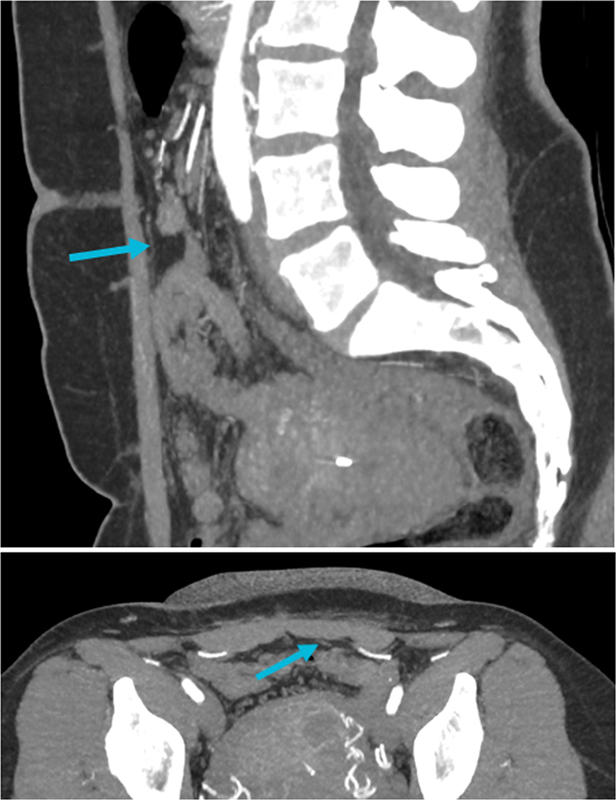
Computed tomographic angiogram (CTA) axial slices demonstrating a peritoneo-cutaneous perforator (blue arrows).
